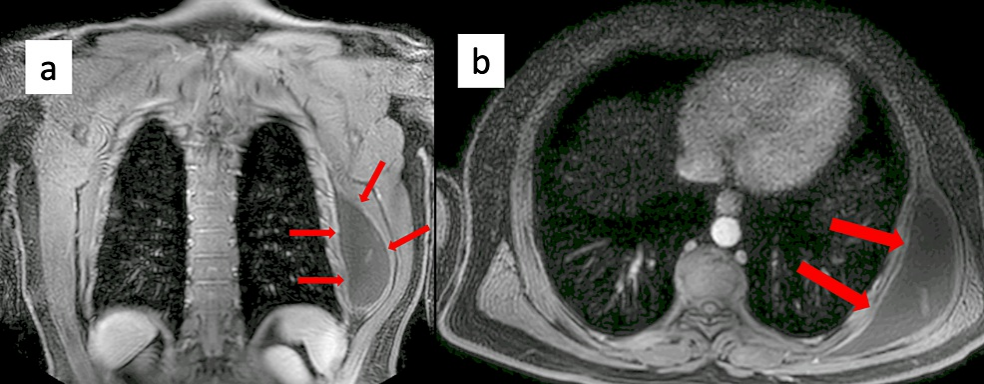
(a) Coronal section and (b) axial section images of giant scapulothoracic bursitis on MRI of thorax.
